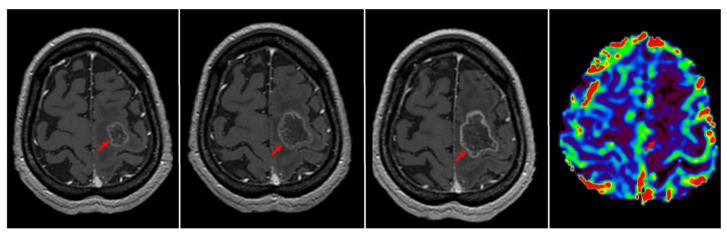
Axial post-contrast T1-weighted MRI of a patient with malignant melanoma brain metastasis treated with stereotactic radiosurgery, acquired at three follow-up time points (19, 23, and 25 months after treatment), showing an irregularly enhancing lesion with progressive dimensional enlargement and central necrotic components (arrows). The relative cerebral blood volume (rCBV) map from the final follow-up examination demonstrates no focal areas of increased perfusion within the lesion, supporting the diagnosis of radiation-induced necrosis despite interval size enlargement.
